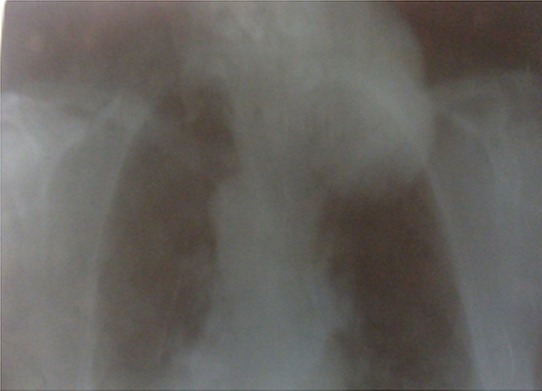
Radiographie du thorax: élargissement du médiastin antérieur avec déviation de la trachée vers la gauche
